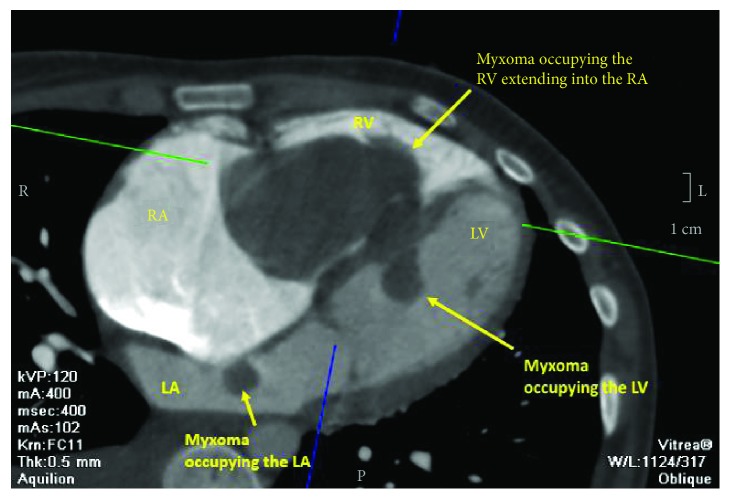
Computed tomography showing a large myxoma occupying the right ventricle and atrium, another myxoma occupying the left atrium, and a third myxoma occupying the left ventricle.
